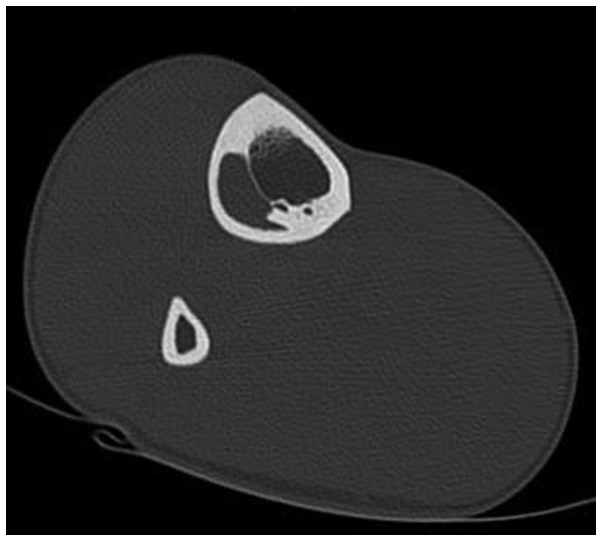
Axial CT image demonstrating an osteolytic lesion in the lateral tibial cortex which did not involve the medullary cavity. CT, computed tomography.
